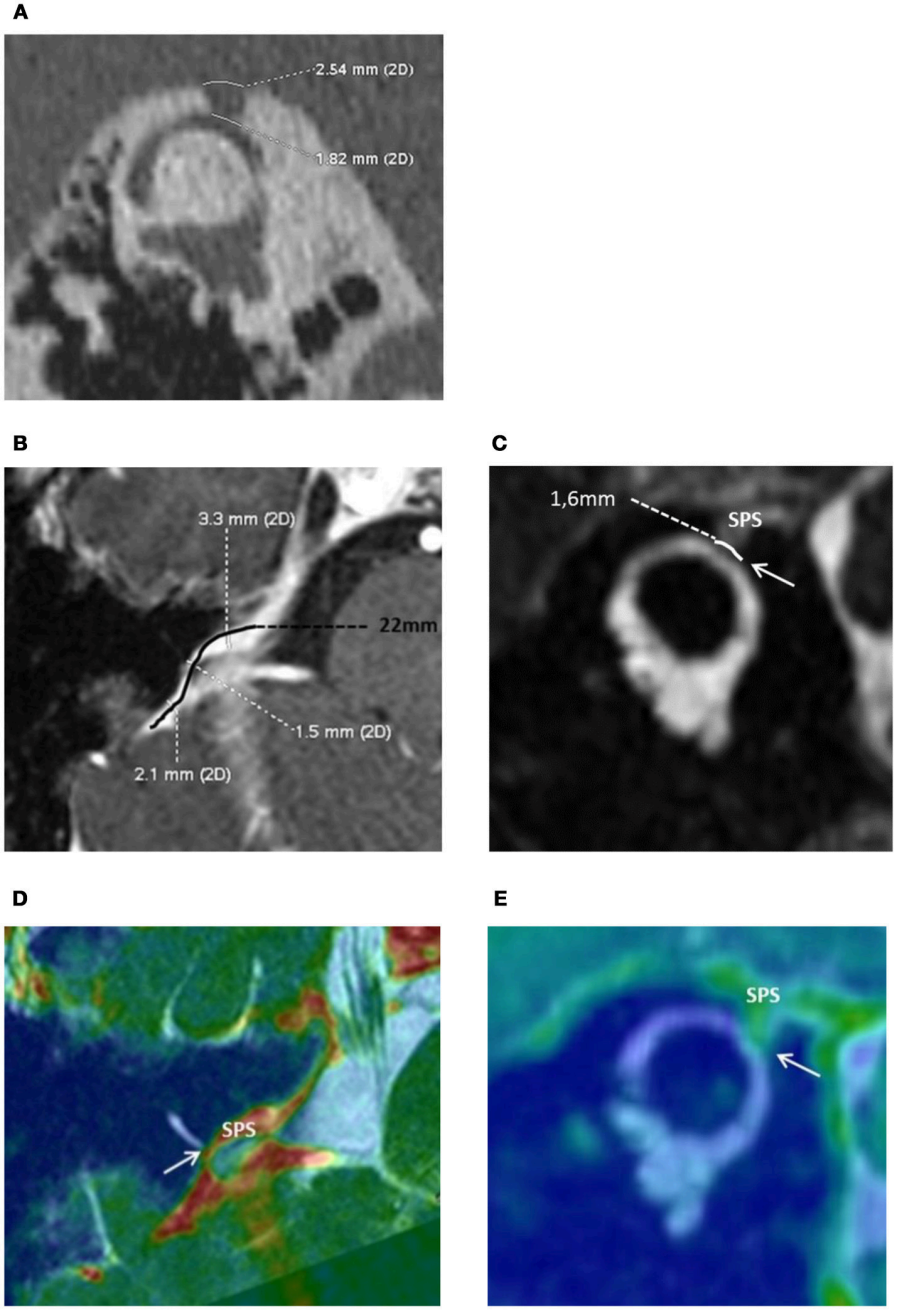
(A) High resolution computed tomography of the right petrous bone. Characteristic “Cookie bite” sign (3) in the Pöschl plane indicating superior semicircular canal (SSC) dehiscence by the Superior Petrosal Sinus (SPS). The bone defect at the dehiscent level between the SSC and the SPS was 1.82 mm in length. (B) Magnetic Resonance Imagery of the right inner ear. The Superior petrosal sinus (SPS) and the Superior Semicircular Canal (SSC): anatomical reports and measurements. Axial 3D T1 weighted enhanced contrast. The length of the right SPS was 22 mm—“S” shape curved black line. Minimal SPS internal diameter in contact with the SSC was measured at 1.5 mm. (C) Coronal oblique T2 high resolution FIESTA, Pöschl plane incidence. SPS wall in contact with the membranous SSC through a limited zone of about 1.6 mm (white arrow). Merged, 3D T1 weighted enhanced contrast and FIESTA axial (D) and coronal oblique sequences (E).
